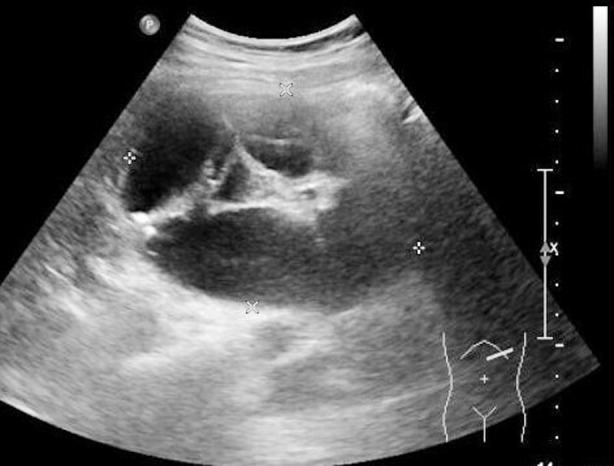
Ultrasonography shows dilated fluid-filled loops of bowel within a herniating mass.
